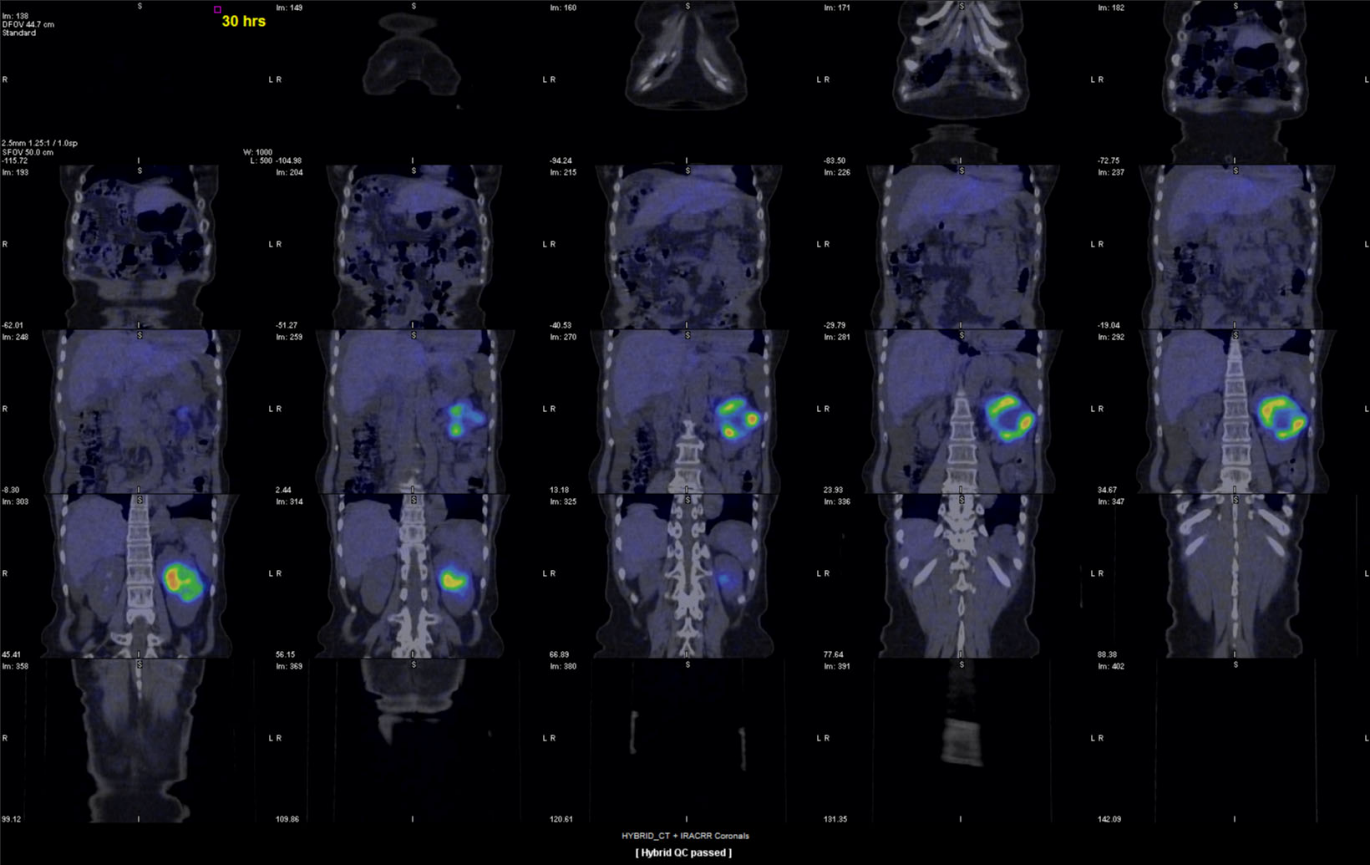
^123^I‐MIBG scintigraphy. ^123^I‐MIBG intake is increased in the left kidney tumor.
